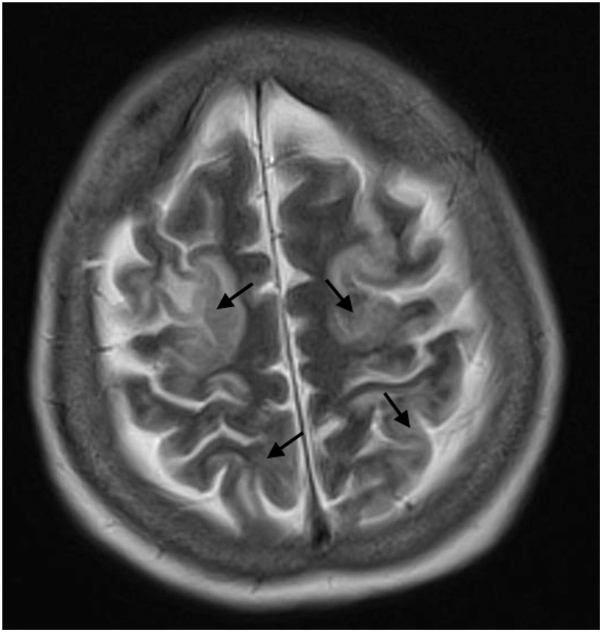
Brain magnetic resonance imaging (MRI) showing extensive T2-weighted hyperintensities involving both cortical/subcortical cerebral regions (*black arrows*).
